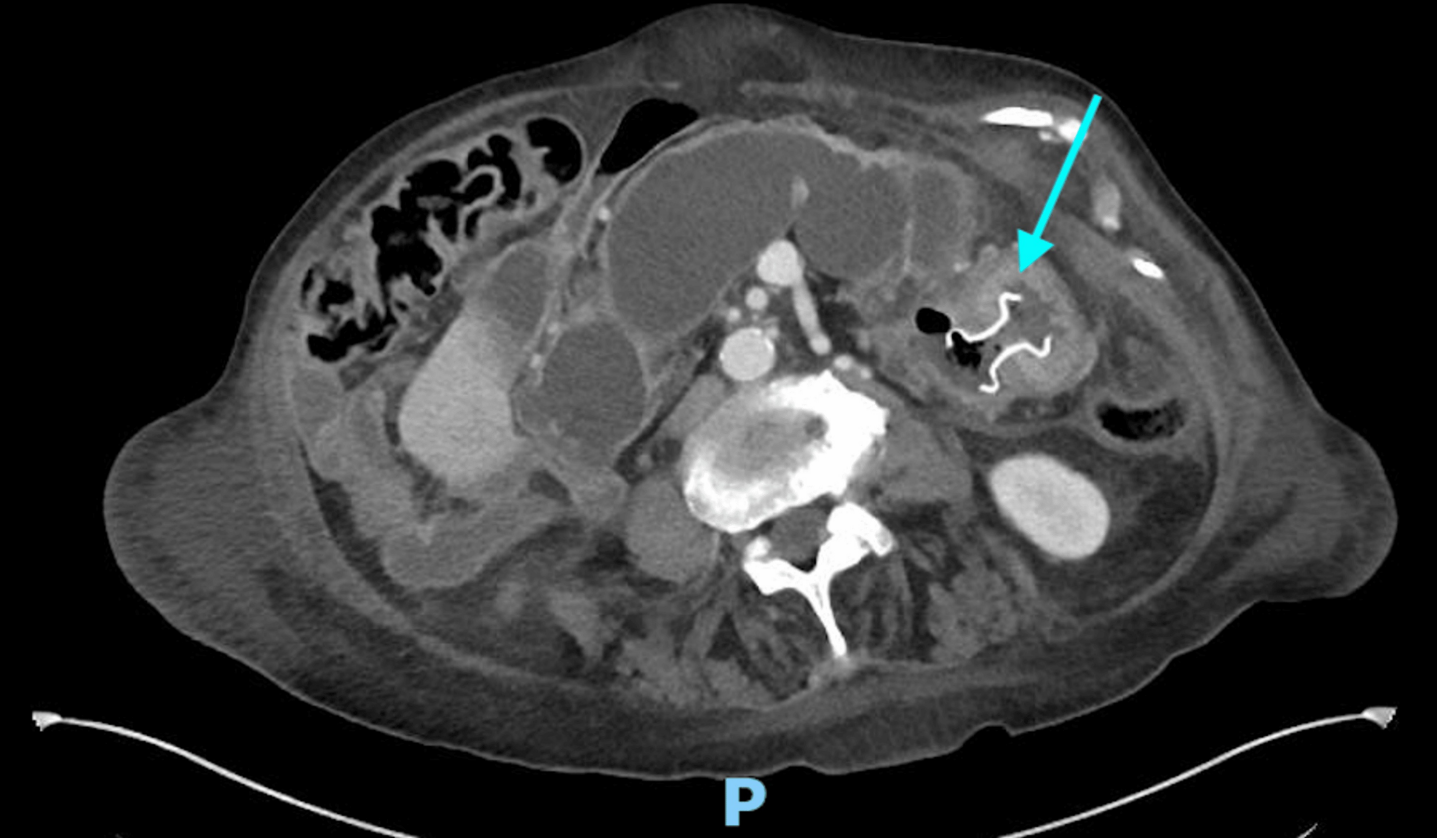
CT of the abdomen and pelvis with intravenous contrast after the placement of the AXIOS stent. CT: computer tomography.
